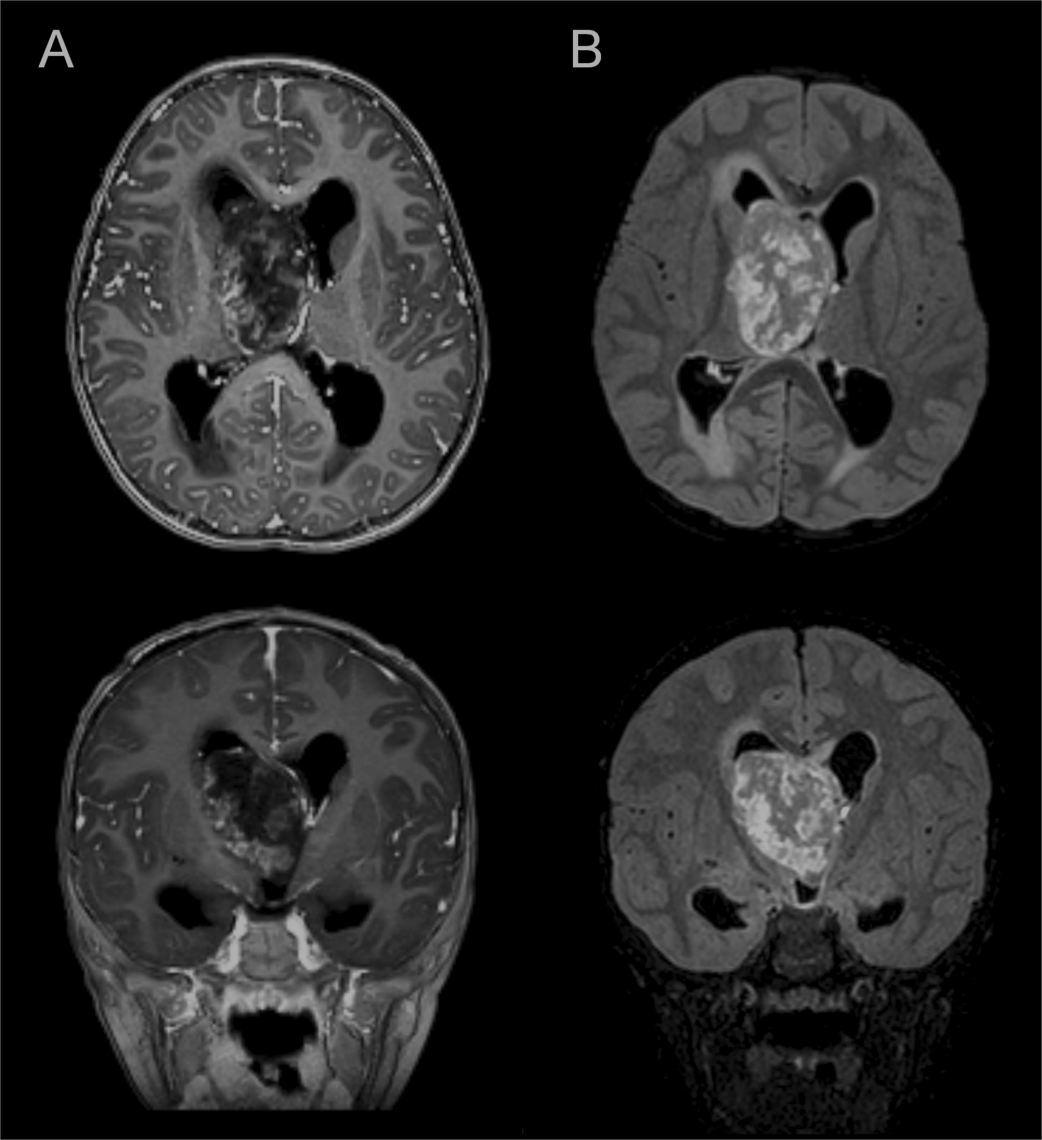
MR study. Contrast‐enhanced T1 weighted (A) and FLAIR (B) images on axial and coronal planes show a large tumour with endoventricular growth, close to the right caudothalamic groove; it shows heterogeneous structure with areas of hyperintense signal in FLAIR and patchy contrast enhancement. The lesion causes severe hydrocephalus due to obstruction of the foramina of Monro, with signs of intracranial hypertension and transependymal oedema.
